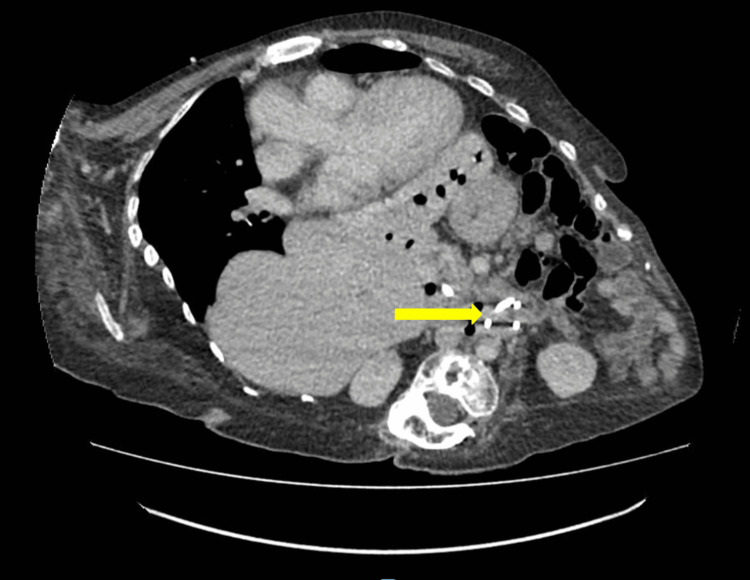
Contrast CT showing the second pigtail stent in the duodenum (yellow arrow)
